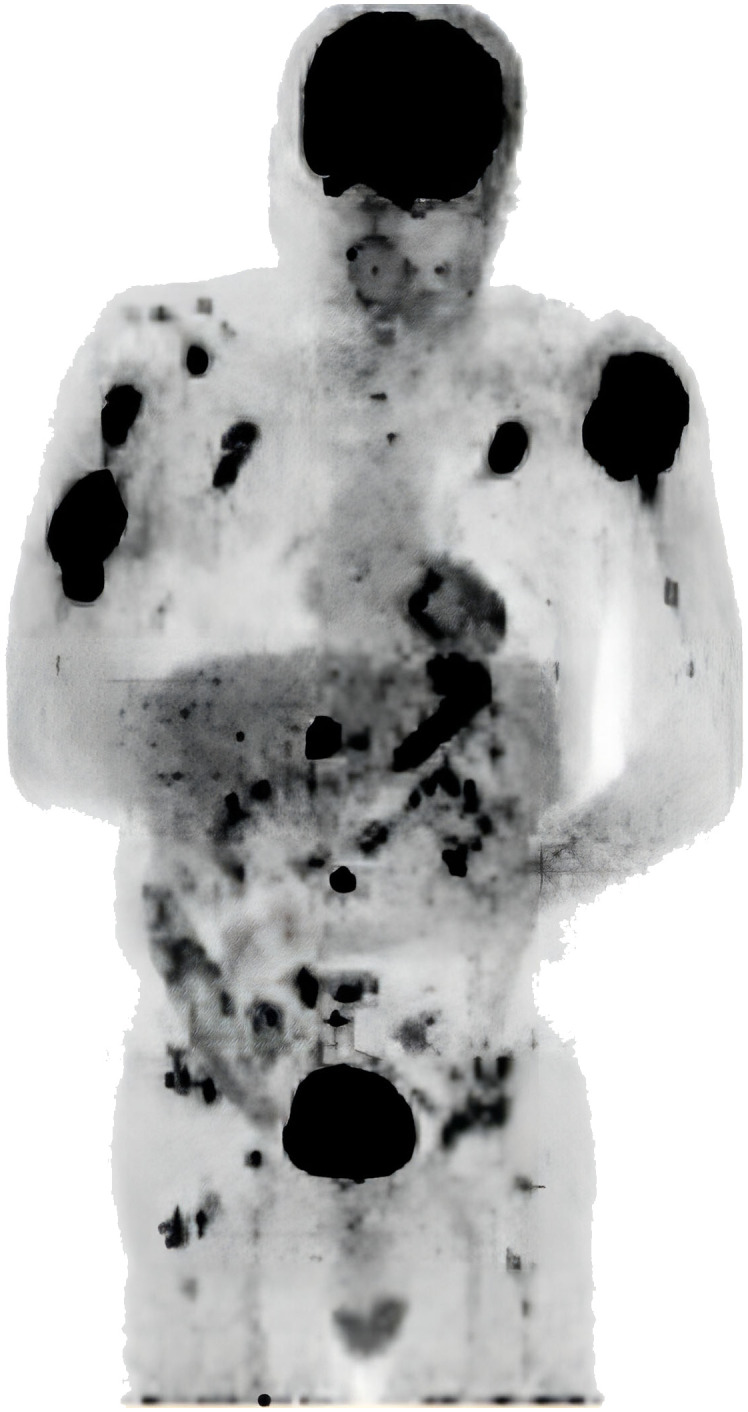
FDG PET/CT showing multiple osteolytic lesions, some lesions accompanied with the formation of soft tissue masses.
